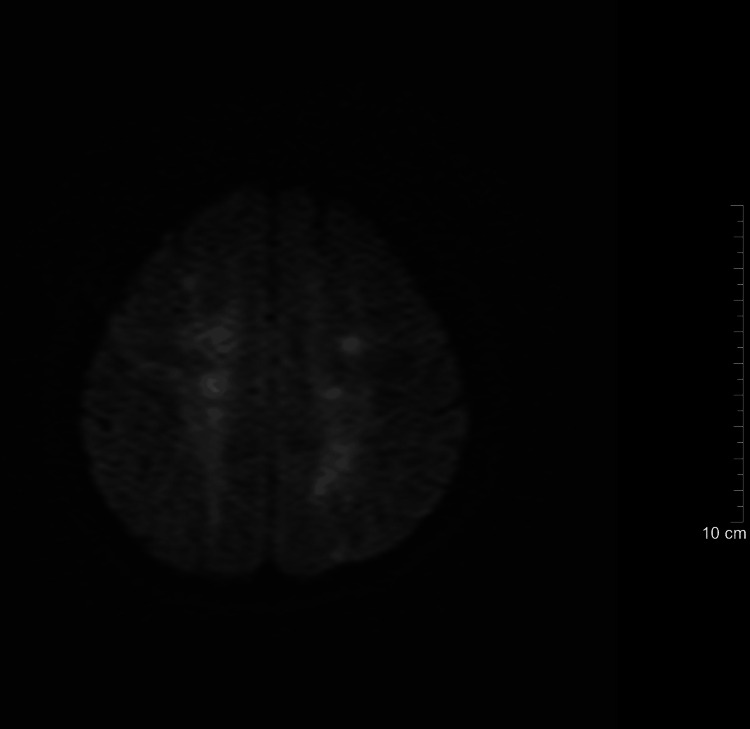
Post-treatment brain MRI (diffusion-weighted imaging, Day 7)
